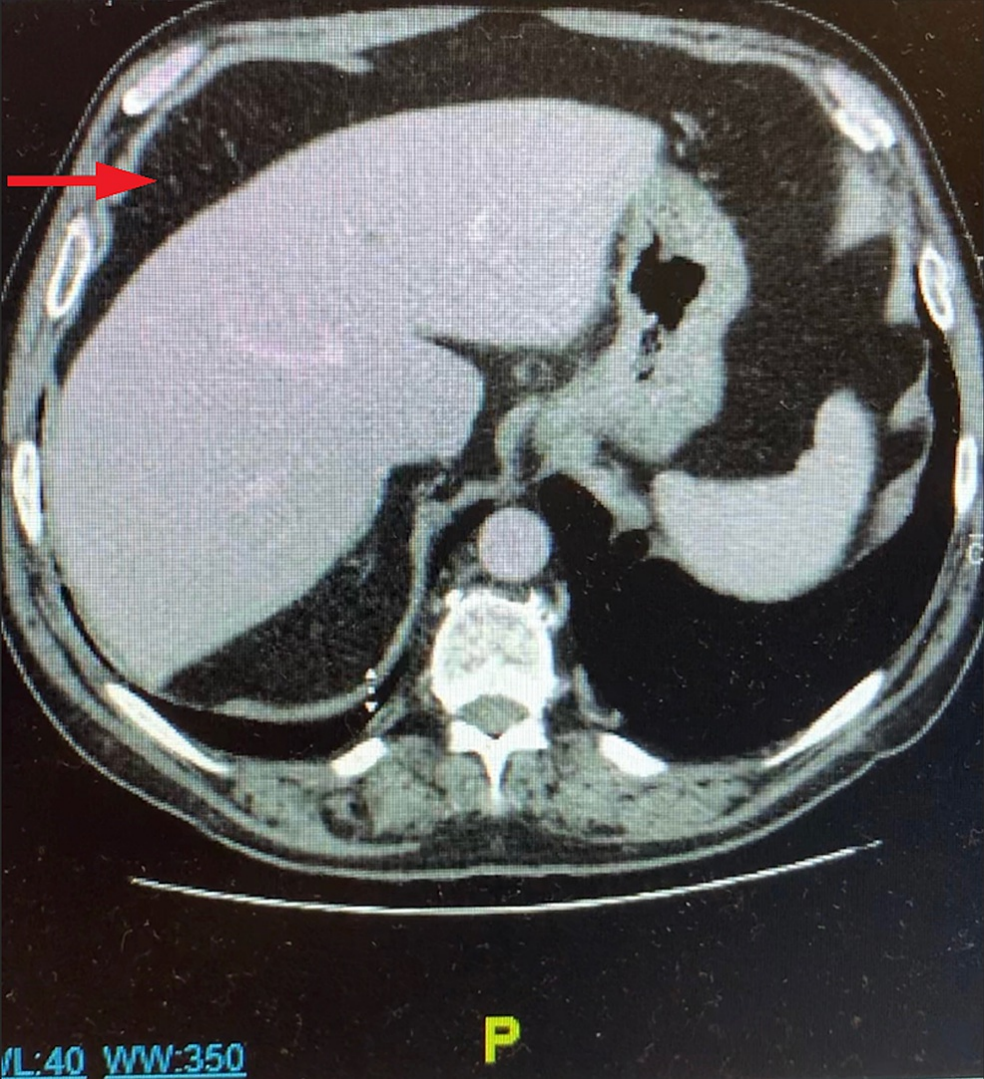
Transverse computed tomography scan without contrast showing colonic interposition between the liver and diaphragm (red arrow).
